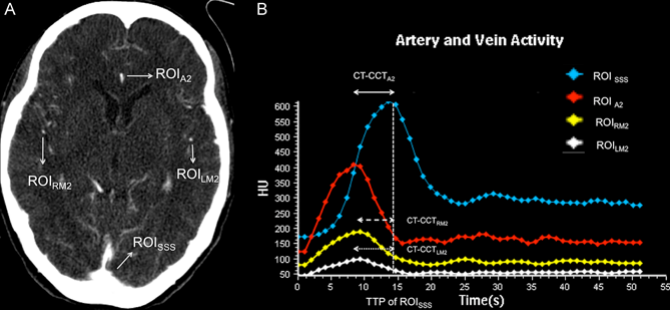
Axial view of the time-maximum intensity projection CTP and the time density curve of ROI. (A) An axial view of the time-maximum intensity projection CTP at the level where the ACA curved anteriorly and posteriorly. ROI_A2_ was chosen at the dominant ACA; ROI_RM2,_ ROI_LM2,_ and ROI_SSS_ were designated at the corresponding vessels. (B) Time density curve of ROI_A2,_ ROI_RM2,_ ROI_LM2,_ and ROI_SSS._ The dashed line intersected perpendicularly with the X-axis indicated the TTP of SSS. The CT-CCT_A2_ (double headed line) was defined as the time difference between the TTP of ROI_A2_ and that of ROI_SSS_; The CT-CCT_RM2_ (dashed double headed line) was defined as the time difference between the TTP of ROI_RM2_ and that of ROI_SSS_. The CT-CCT_LM2_ (dotted double headed line) was defined as the time difference between the TTP of ROI_LM2_ and that of ROI_SSS_.
